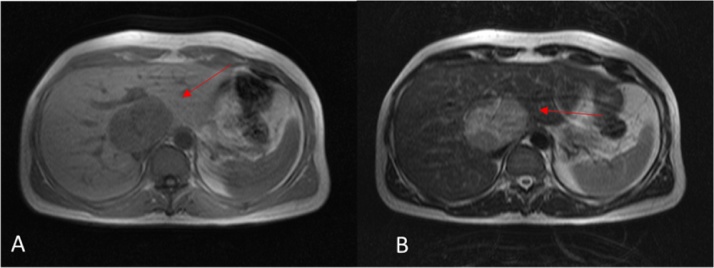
MRI of the abdomen. A well-marginated mass appears as a hypointense area on T1-weighted images (A), and hyperintense area on T2-weighted images (B).
